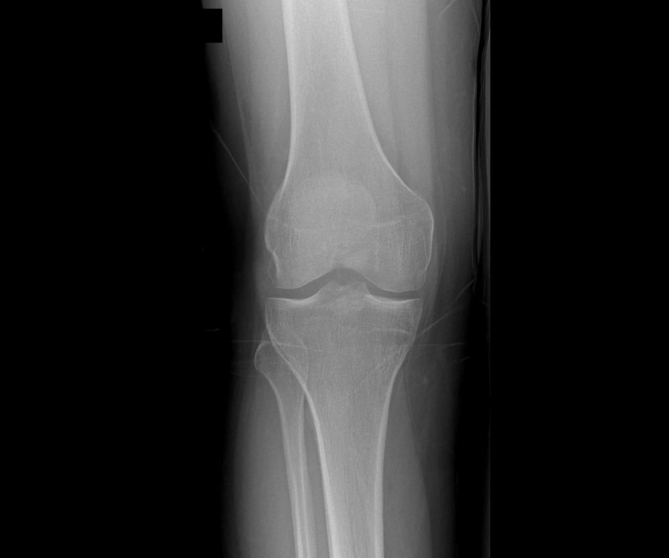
The integrity of the joint and donor site is verified on a preoperative radiograph of the knee.
